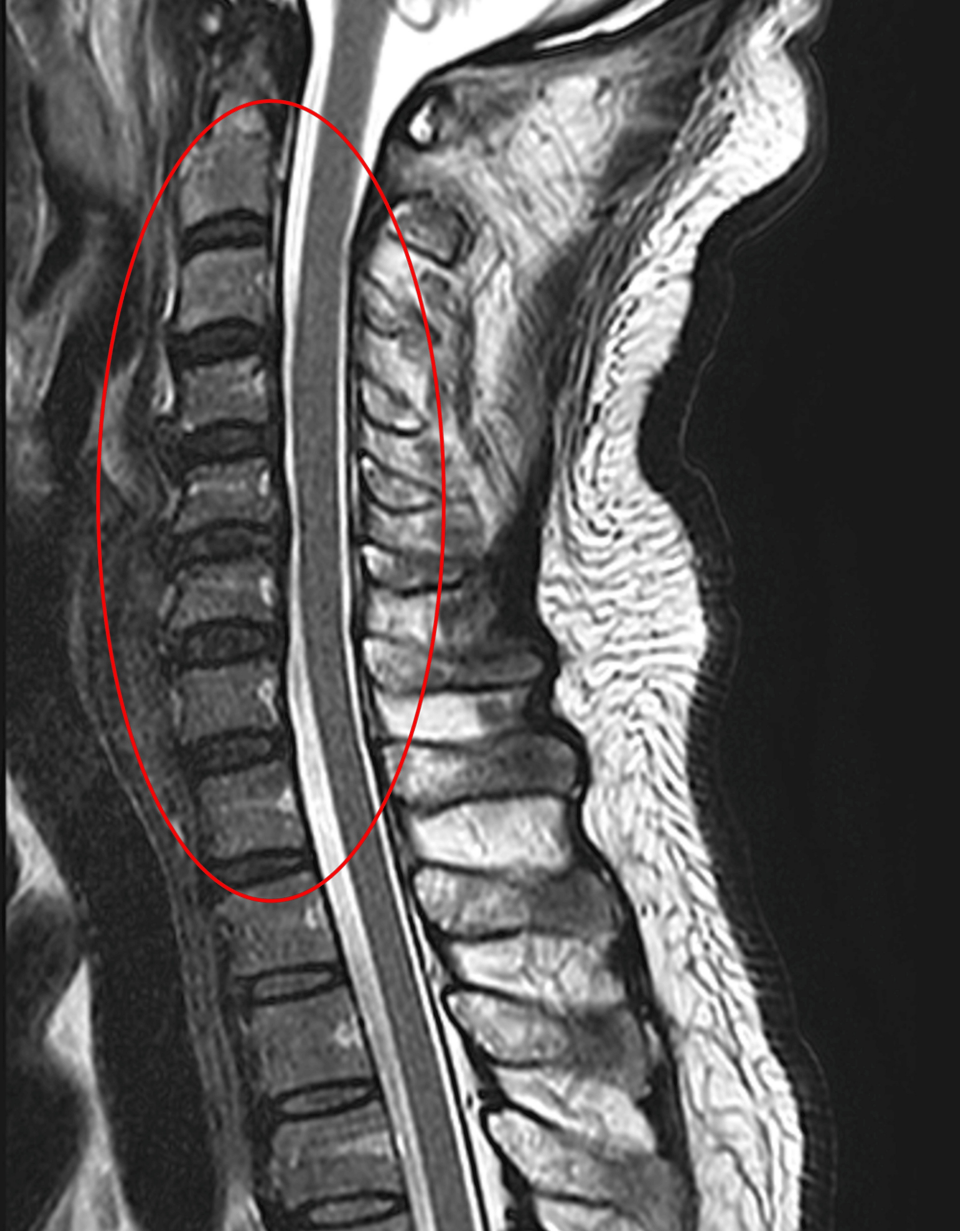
Sagittal T2-weighted MRI of the cervical region (circled) demonstrating the absence of intrinsic or extrinsic spinal cord abnormalities MRI: magnetic resonance imaging
